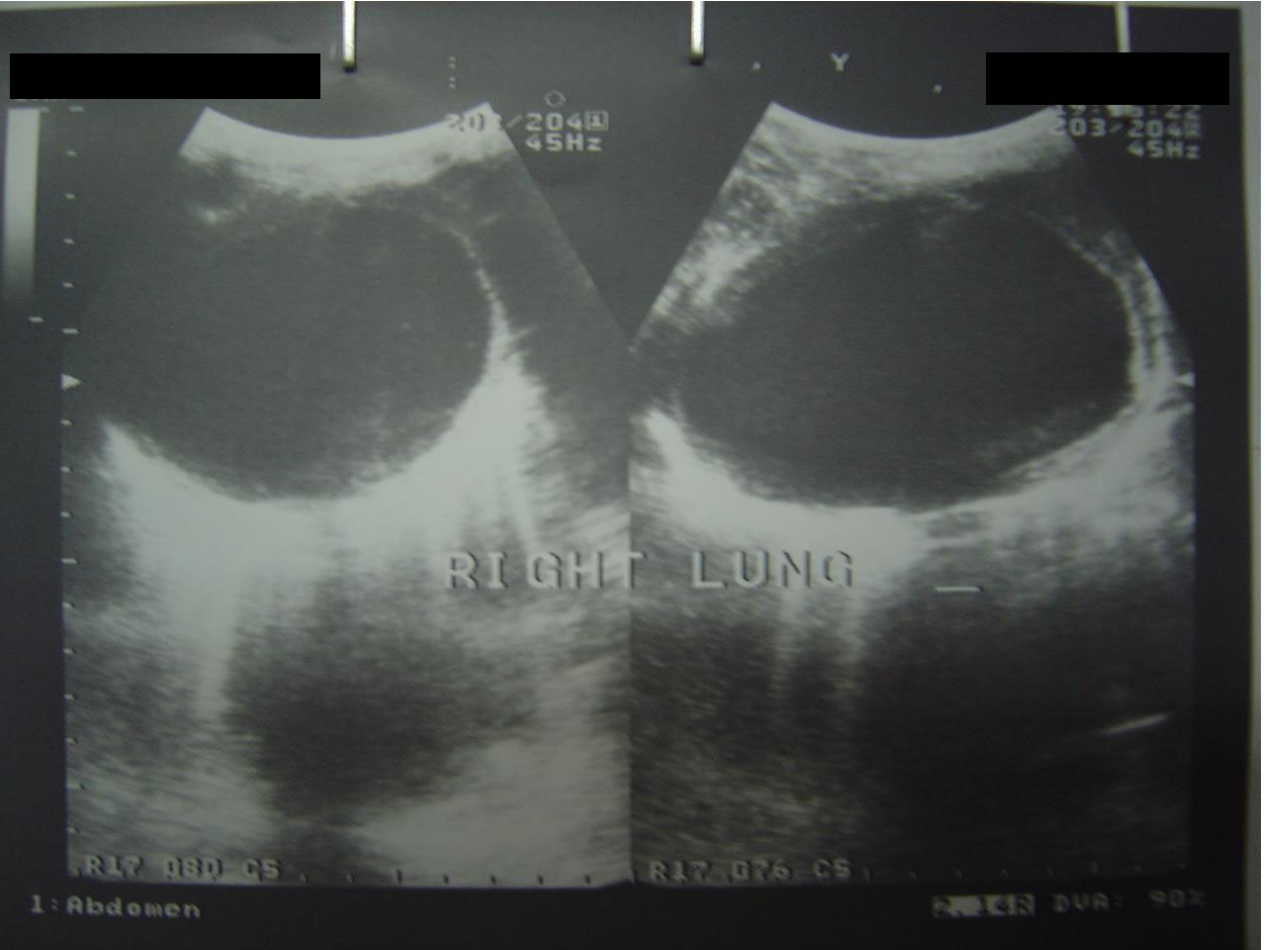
Case 1 – Ultrasound of right lung shows a large cystic lesion with a with the double wall sign.
